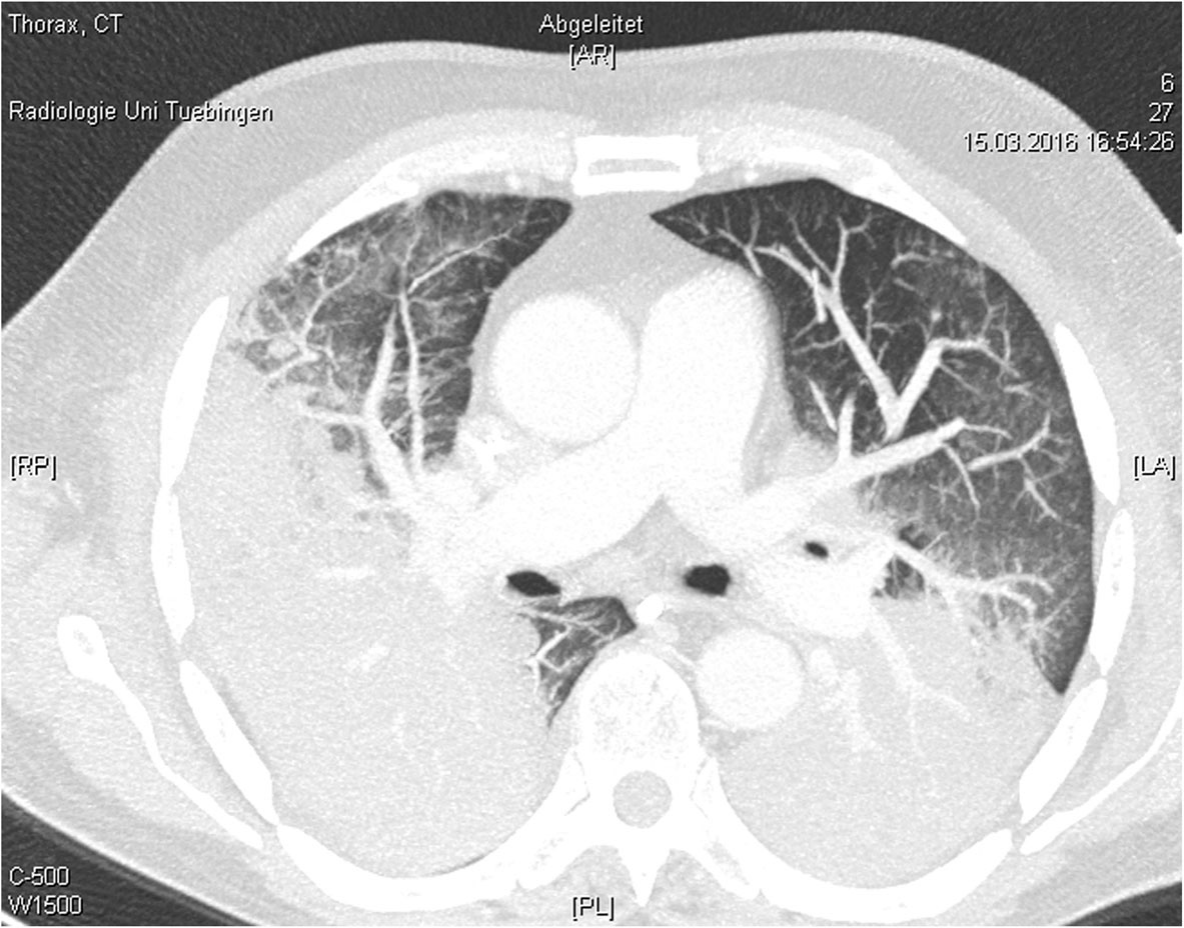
CT scan of the lung on day 1 after admission
